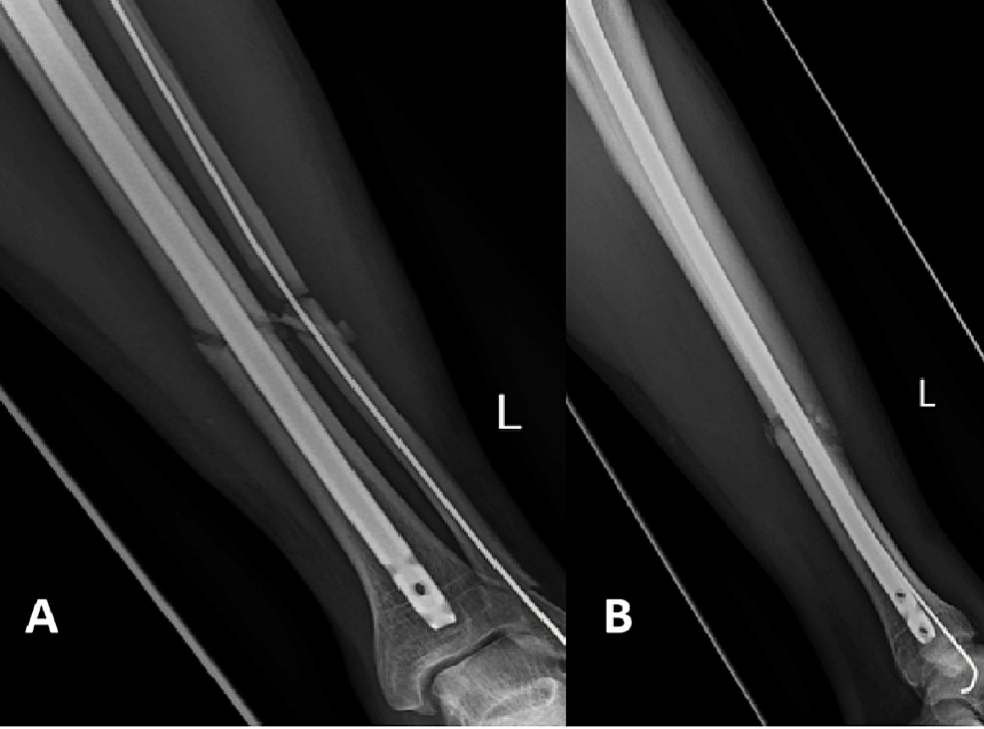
Anteroposterior (A) and lateral (B) radiographs of a tibia/fibula fracture after three months with radiological features of delayed union
